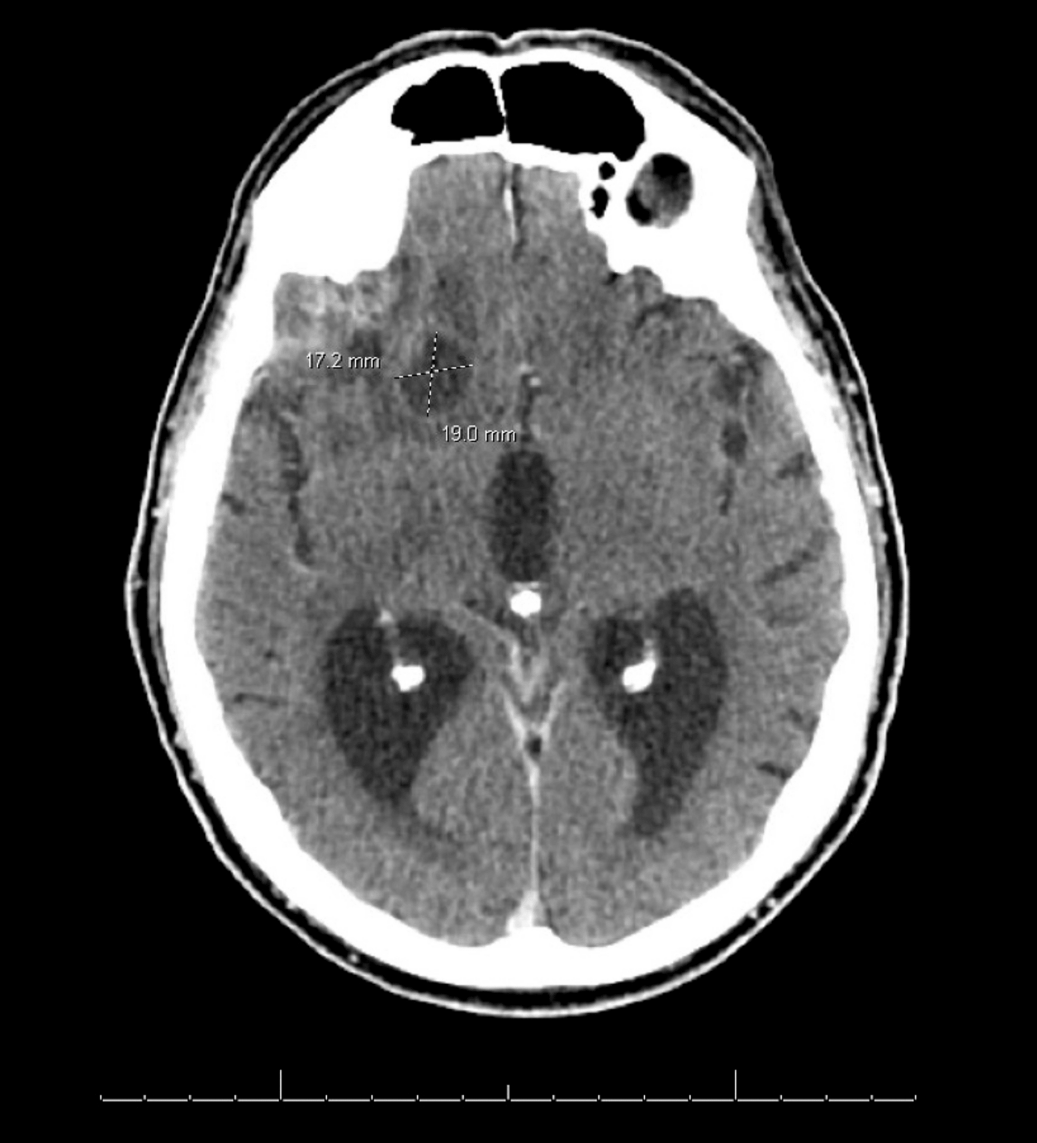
CT scan of the brain showing the right frontal lobe lesions. The scan demonstrates a low-attenuation lesion in the right frontal lobe (1.9 x 1.7 cm) with heterogeneous enhancement. No mass effect is noted. CT: Computed tomography
